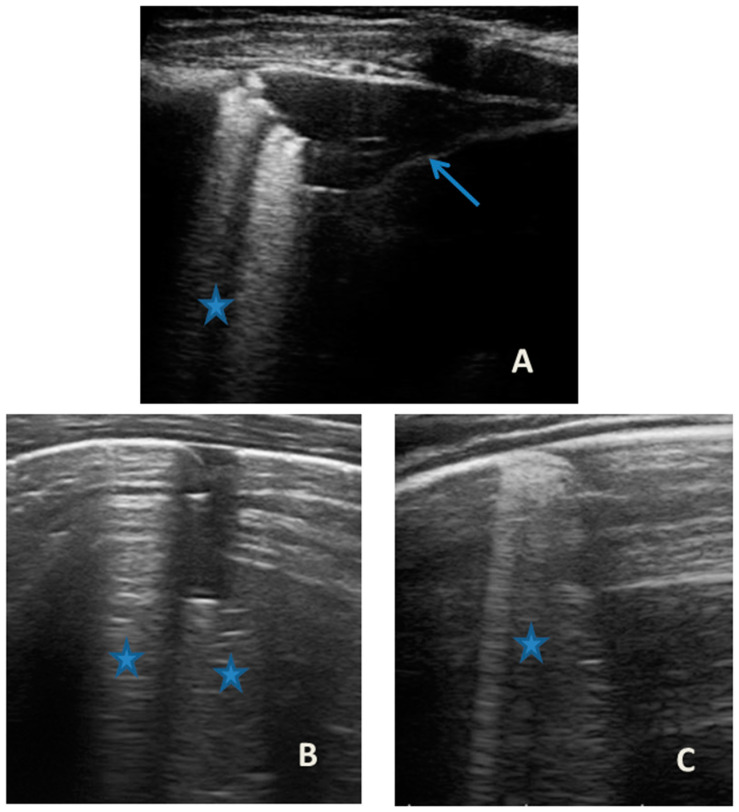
Grayscale lung ultrasound examination shows: an irregular pleural line in all fields explored; on the right anterior field, mainly in the medio-apical area, in the paracardiac area (A); and on the right anterior field in the basal area (B); consolidations of an atelectasis nature (arrow) poorly aerated, associated with sonographic interstitial syndrome (SIS), represented by coalescent vertical artefacts or B-lines and “white lung” areas (asterisks), which are also present in on the bilateral lateral fields (C).
